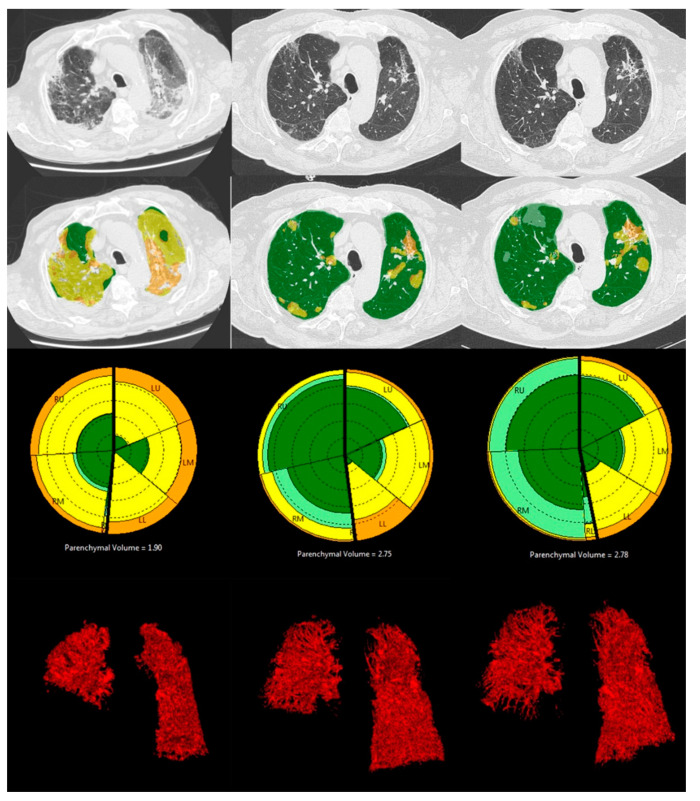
CALIPER analysis of a CT scan of a 92-year-old male patient with right hemidiaphragm elevation and right lower lobe collapse at T0, T12 and T24, from the left to the right. From the upper to the bottom: axial CT slice, axial CALIPER-derived color image overlays (dark and light green = Norm, yellow = GG, orange Ret), the glyph of the lung parenchymal patterns and the 3D volume rendering reconstructions of lung vessels. At T0, the patient presented diffuse areas of GG and Ret. At T12, most of the abnormalities had resolved, while areas of GG associated with Ret persisted, predominantly on the left; these findings persisted also at T24.
